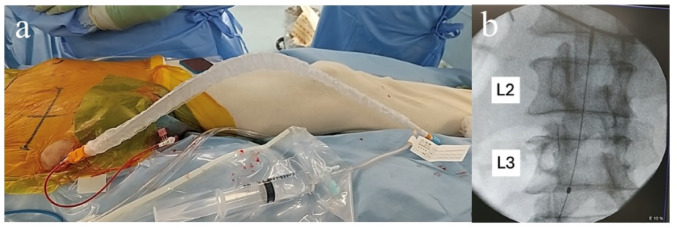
a Percutaneous insertion of the intra-aortic balloon catheter via the contralateral femoral artery under ultrasound guidance. b Fluoroscopic image showing the tip of the intra-aortic balloon catheter positioned in Zone 3 of the aorta, between the renal arteries and the aortic bifurcation
